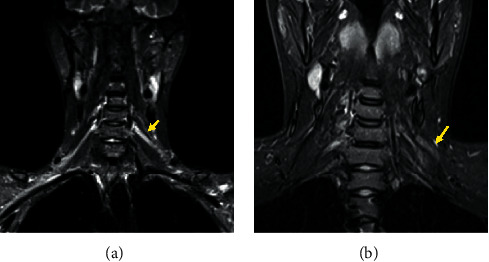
T2 weighted MRI of the brachial plexus showing the onset of the compressive phenomenon after post-traumatic injury, where the left panel shows an initial inflammatory process at an early stage of injury and the right panel image shows the development of fibrosis at a later stage. (a) The arrow points the swelling of the root with increased signals. (b) The image shows a fibrotic process located in the left C5 to C7 brachial plexus trunks (arrow), secondary to the traumatic injury, manifested as pain, symptom explained by the connective tissue that surrounds the nervous structures, generating a compressive phenomenon that originates strangulation of the nerve, inducing hypernociception.
